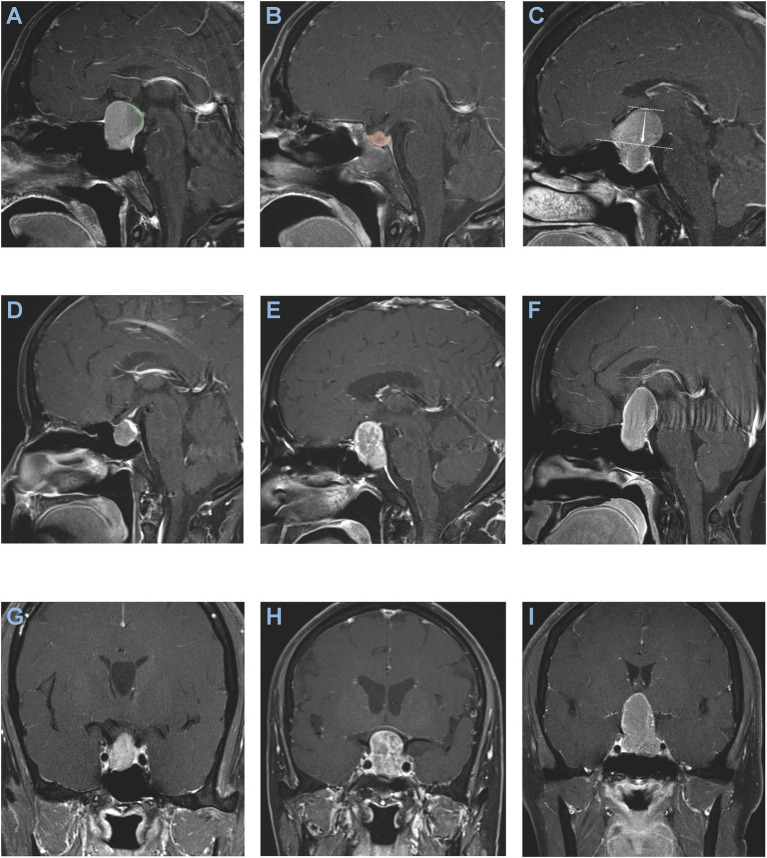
Radiological evaluation. (A) The green filled area indicates the preoperative location of the pituitary gland. (B) The red filled area indicates the postoperative pituitary position. (C) After aligning the MRI and CT images using 3D slicer software, the line connecting the anterior bed and the sella turcica (dashed line below) was obtained, and the vertical distance between the highest point of the tumor and this line was calculated. (D) Pituitary stalk type 1: no displacement. (E) Pituitary stalk type 2: displacement with discernible morphology. (F) Pituitary stalk type 3: indiscernible stalk. (G) Optic nerve type 1: no compression. (H) Optic nerve type 2: compression with recognizable deformation. (I) Optic nerve type 3: indiscernible optic nerve location.
